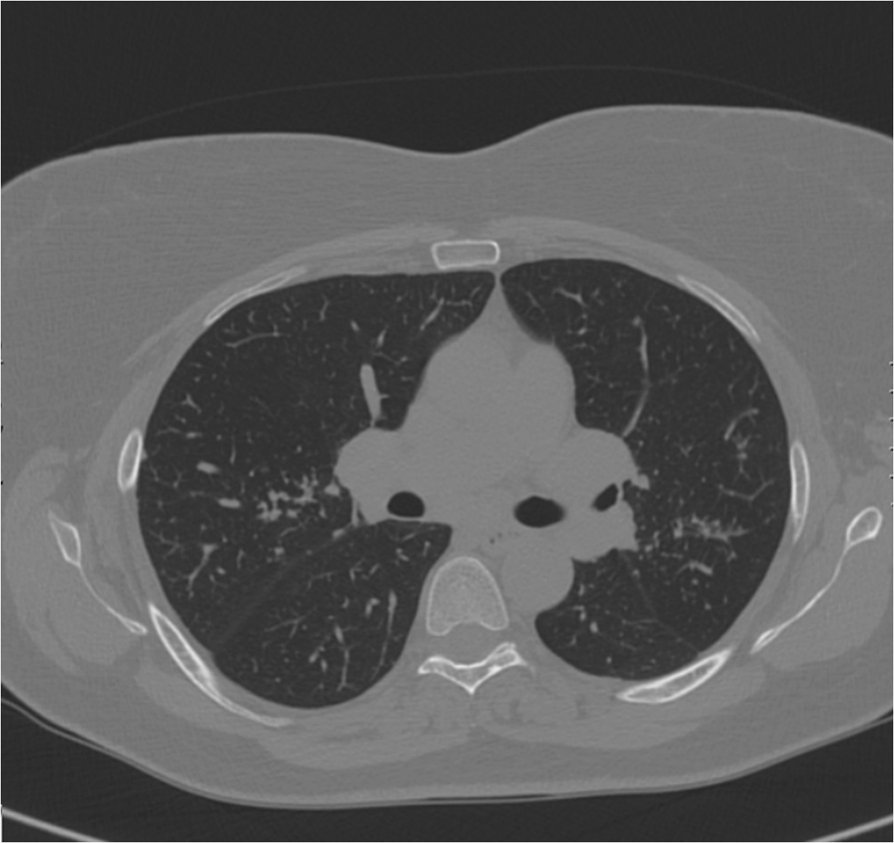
Chest CT scan of the woman showing: “a series of lung nodules distributed in all lobes”.
